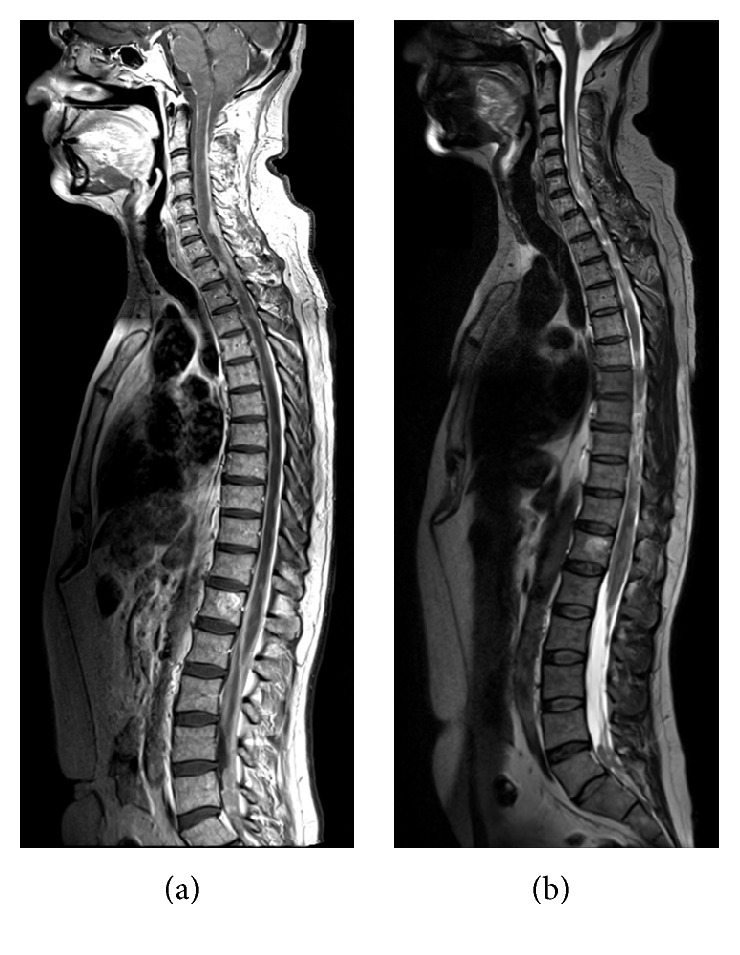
Contrast-enhanced T1- (a) and T2-weighted (b) whole spine MRI sequences showing disseminated intradural intra- and extramedullary Embryonal Tumor of the cervicothoracic tract and cauda equina.
